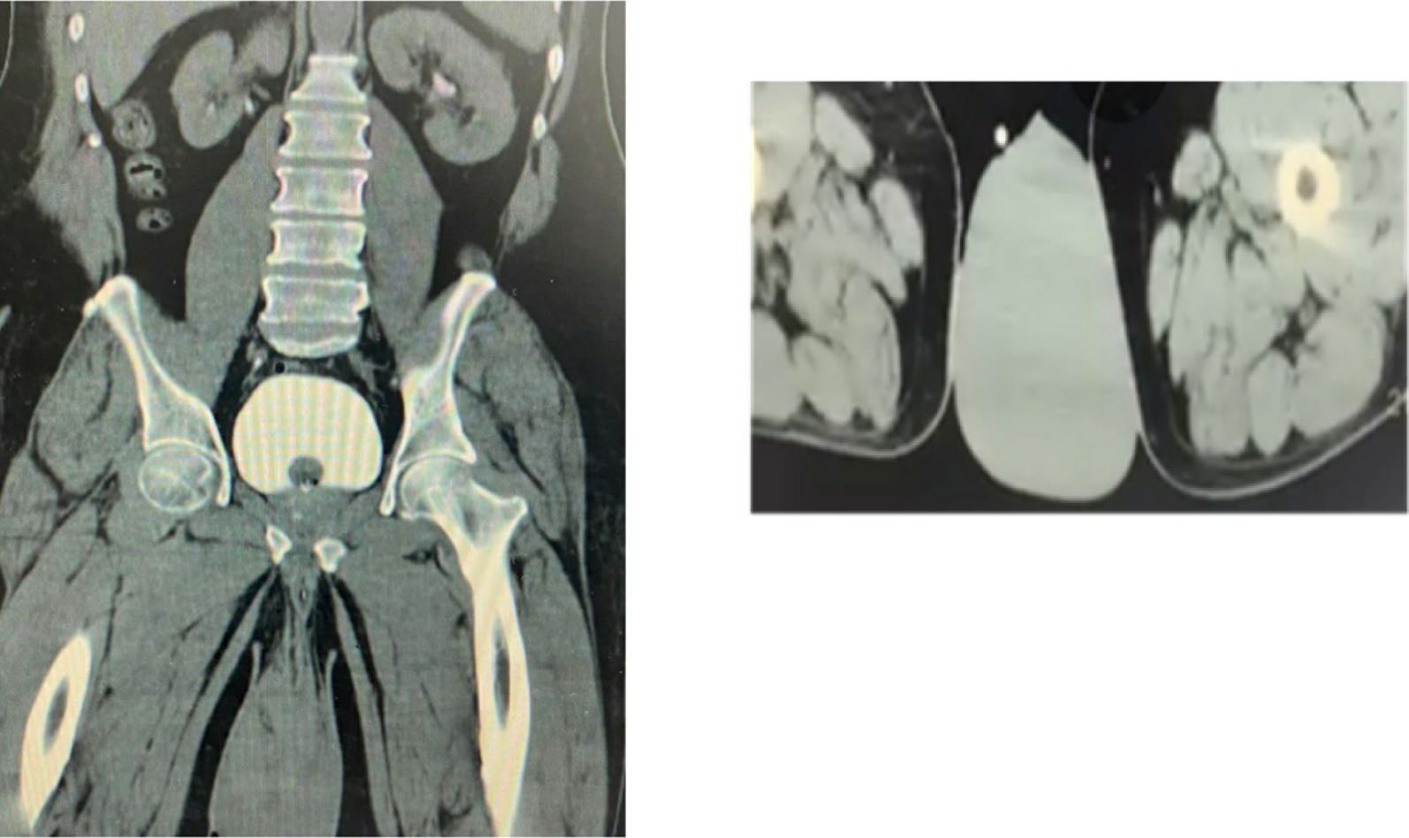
CT scan of the abdomen and pelvis; big scrotal hematoma around the right testis with stranding of the fat tissue and thickening of the soft tissue planes at the right inguinal region. No retroperitoneal hematoma
